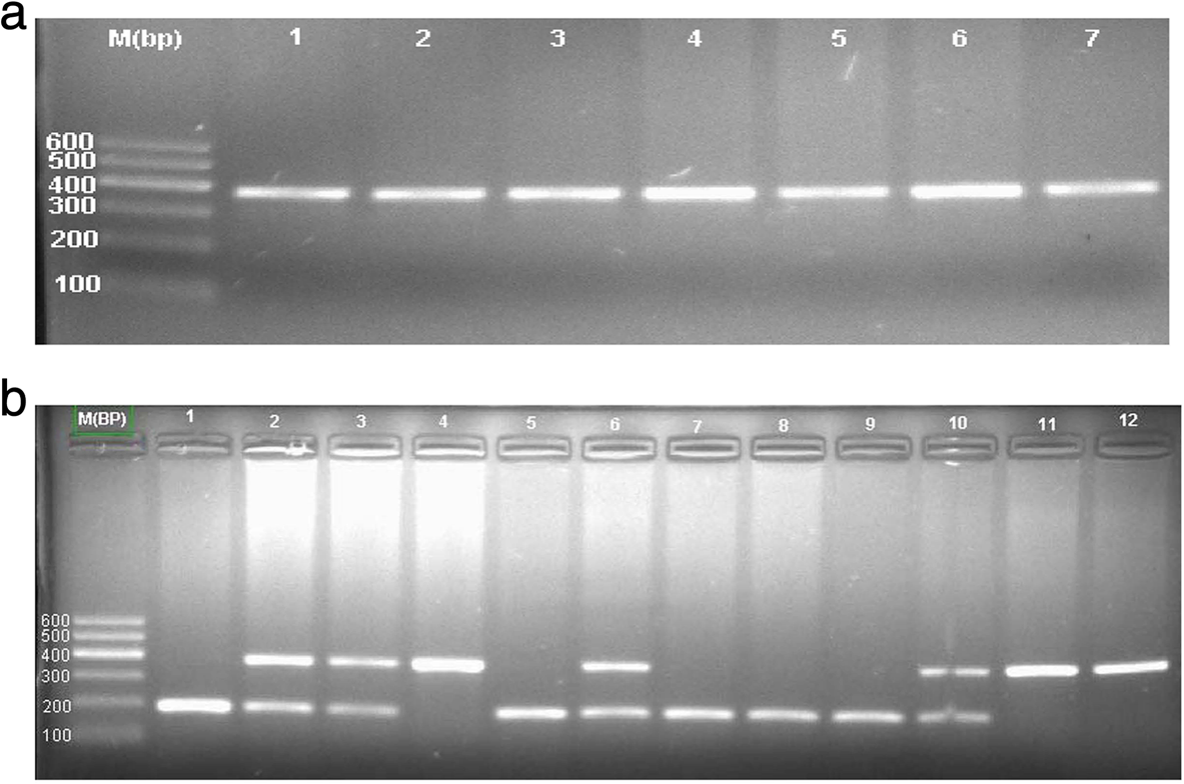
The PCR and digestion products of rs2592551. a: rs2592551 PCR product with product length of 381 bp; b: rs2592551 digestion products. 2, 3, 6, 10 were heterozygous CT genotype. 1, 5, 7, 8, 9 were mutated homozygous TT genotype. 4, 7, 8, 9 were homozygous CC wild-type (Due to the 189 and 192 bp enzyme digestion lengths were very close to each other, there showed overlap on the electropherogram showing as one band).
